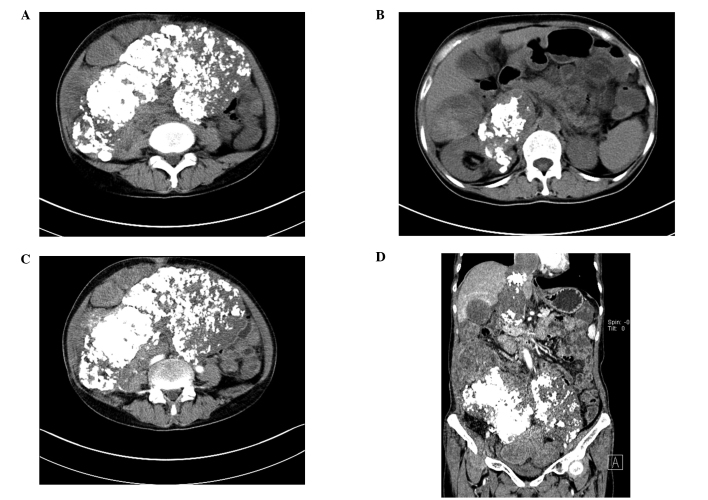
(A and B) Unenhanced transverse CT reveals heterogeneous masses with extensive dense, as well as ring- and arc-like calcifications in the retroperitoneum and inferior vena cava. (C) Enhanced transverse CT reveals subtle heterogenous enhancement in the periphery of the mass, but no enhancement is evident in the majority of the mass. (D) Enhanced coronal CT shows a clear mass in the inferior vena cava. CT, computed tomography.
